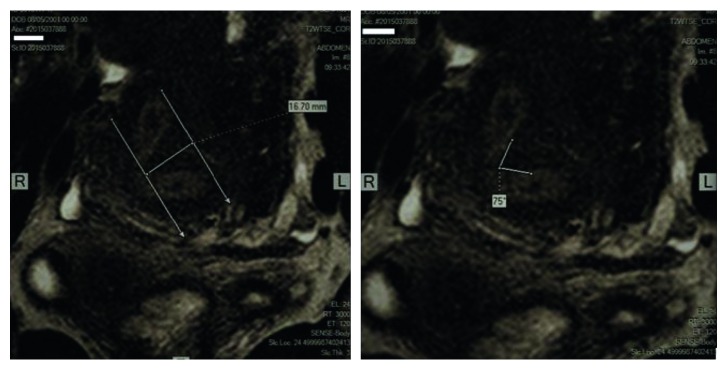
MRI and coronal view of the septate uterus. Internal indentation depth is 16.7 mm and the indentation angle is 75°, with a normal external fundal contour, and these measurements are in agreement with the definition for septate uterus by the ASRM and the CUME [7, 8].
